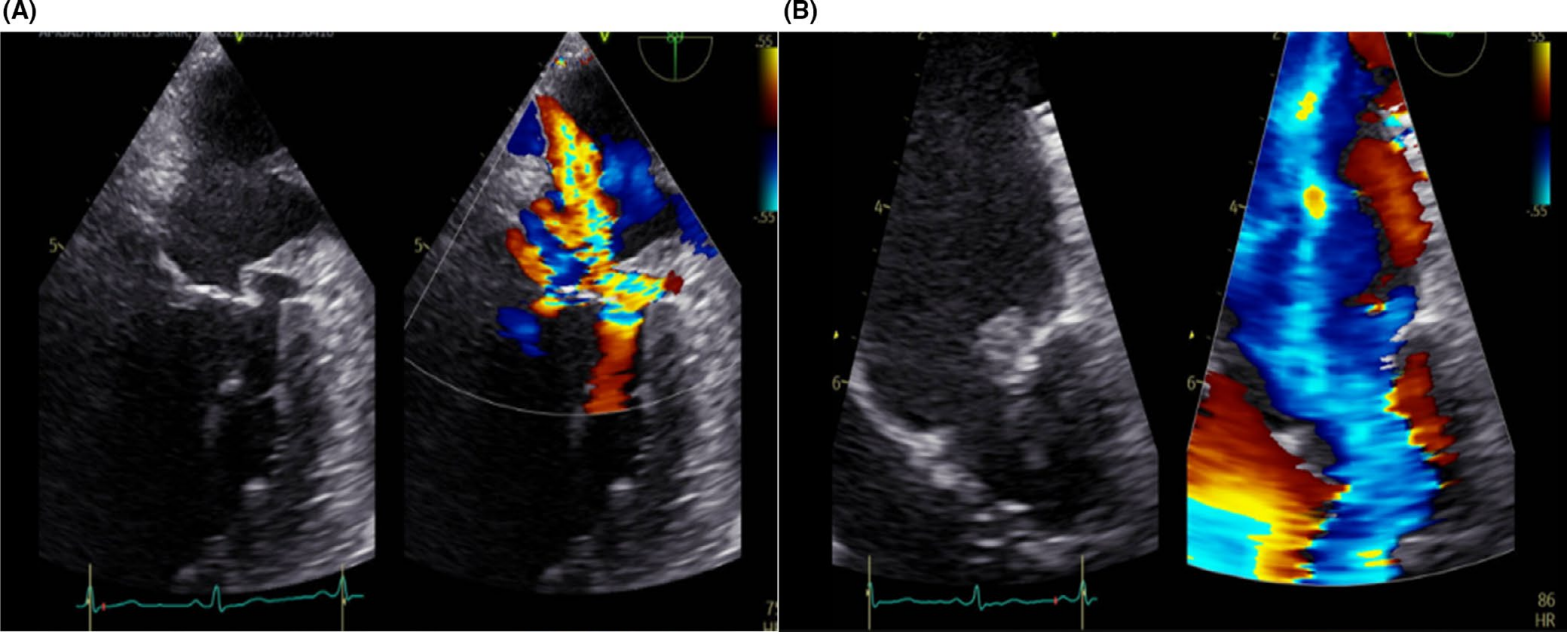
(A and B) Transesophageal echocardiography revealed a perforated posterior mitral valve leaflet with abscess formation and severe mitral valve regurgitation
